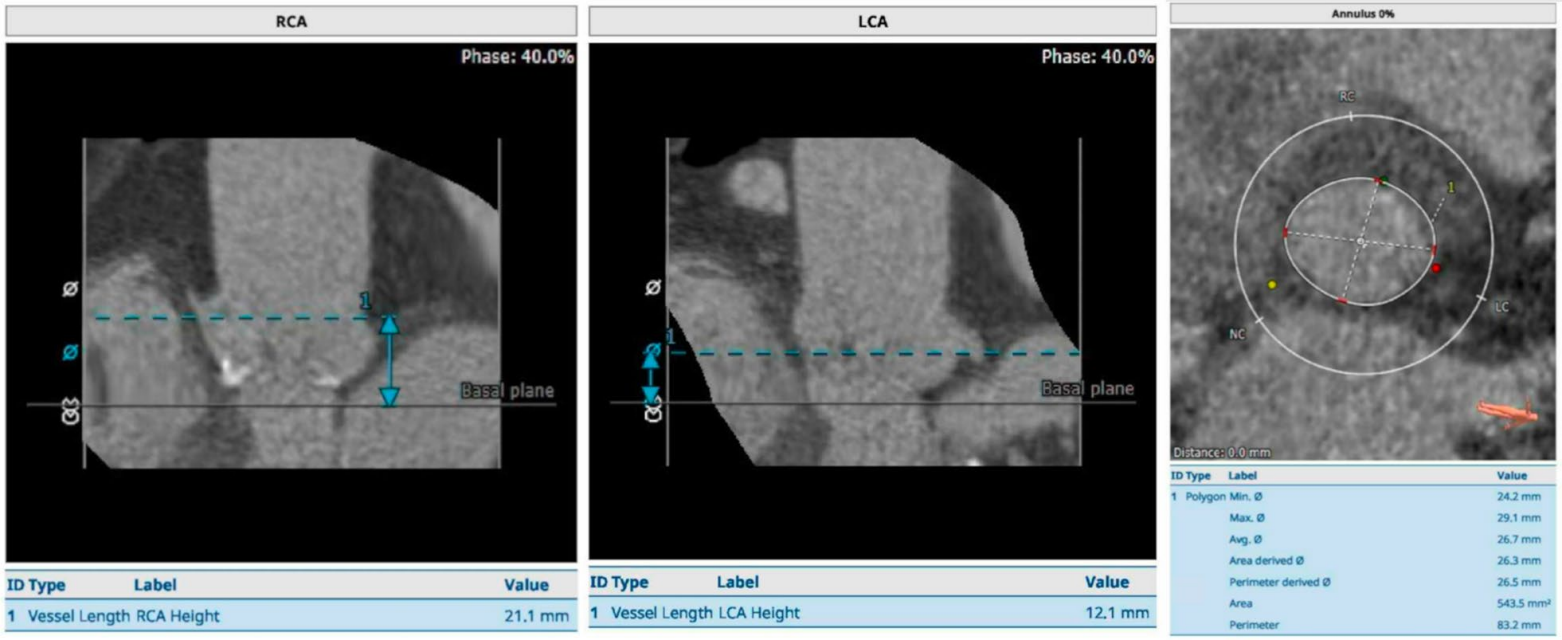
Pre-Operative CT scan showing anatomy of the transplanted aortic valve and root. The left and middle images demonstrate the left and right coronary heights, while the right image demonstrates the dimensions of the annulus
